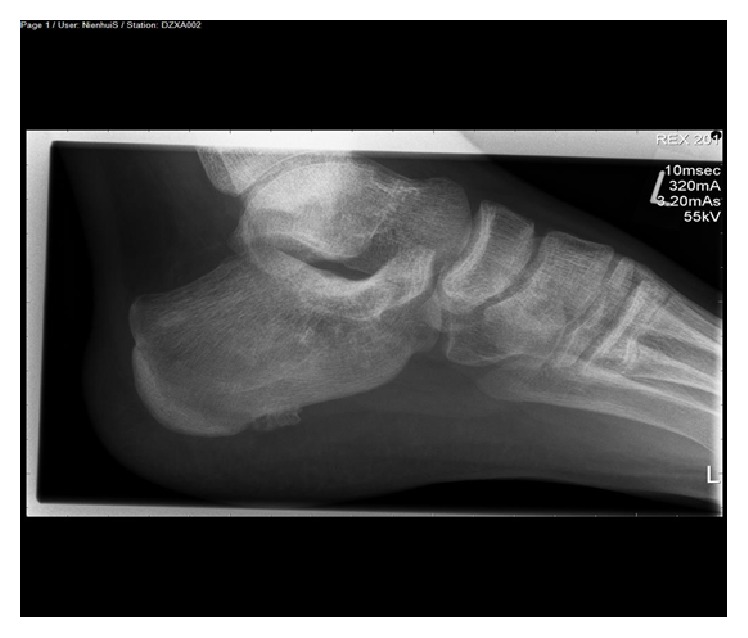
Plain radiograph: lateral view of the left foot: osteochondroma located at the plantar side of the calcaneal bone.
